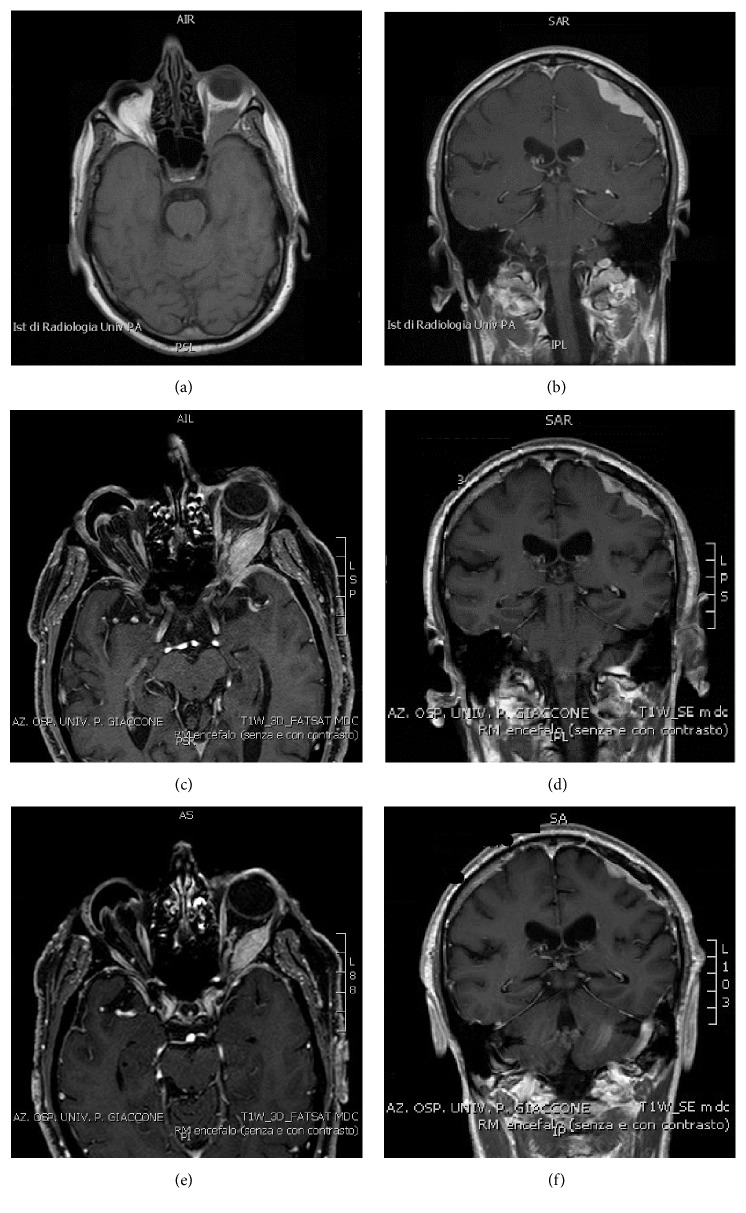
Axial (a, c, e) and coronal (b, d, f) enhanced T1 MR images reveal enhancing masses along the left frontoparietal-temporal region and the orbital region with dislocation of the optic nerve at onset (a, b) and in one-year (c, d) and two-year (e, f) follow-up, respectively.
